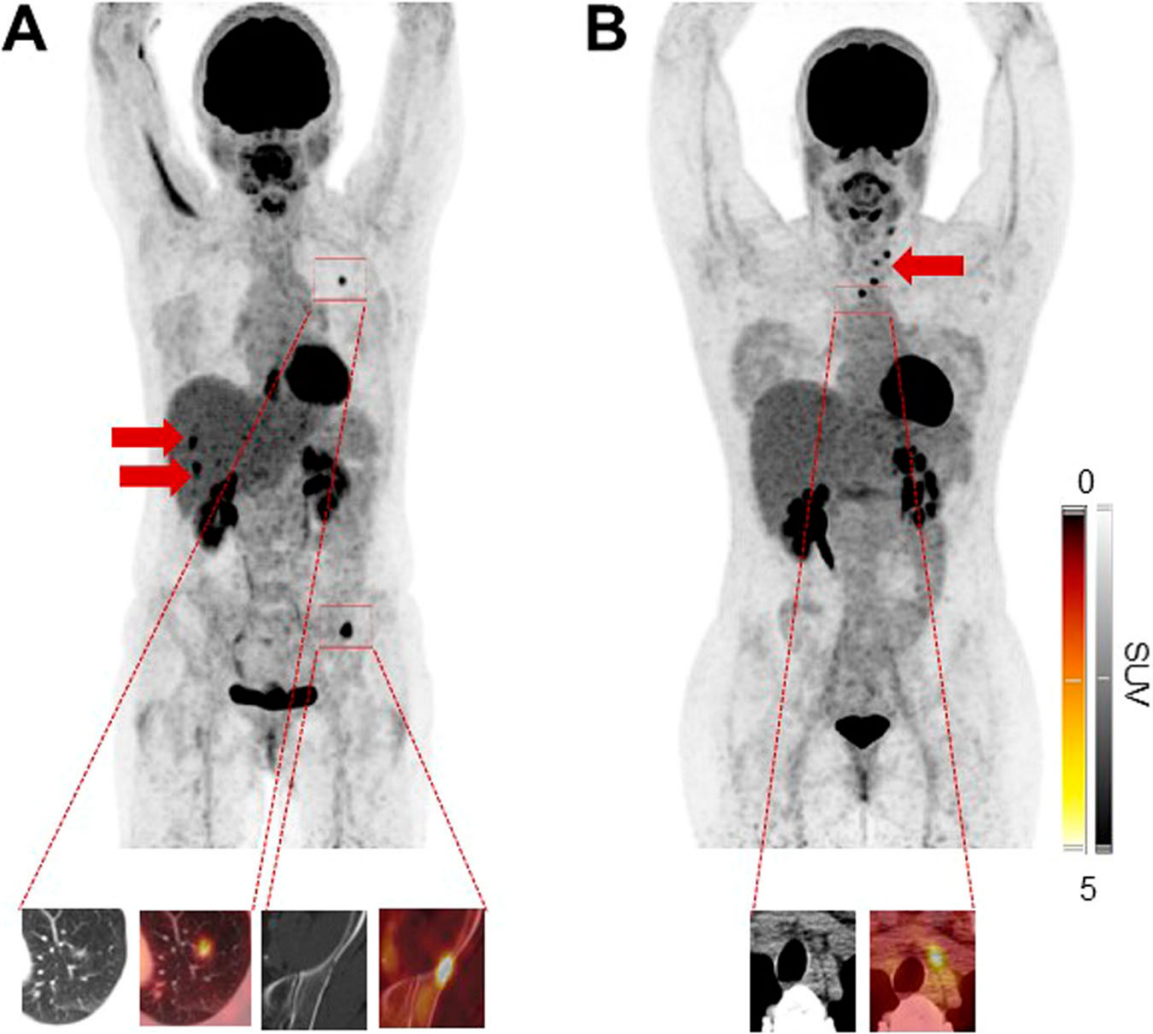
Patients with management changes after [^18^F]FDG PET/CT, with (A) systemic and (B) non-systemic treatment alterations. Maximum intensity projections and transaxial CT and PET/CT portions of target lesions are also displayed. Subject in A showed multiple organ involvement with PET-positive pulmonal and osseous findings (in the left pelvis) displayed on transaxial CT and PET/CT. Of note, lung lesions were only identifiable in the PET/CT images. Additional PET-positive hepatic lesions are not shown on transaxial slides, but seen on the MIP (arrows). Given widespread disease revealed by [^18^F]FDG, this patient was scheduled for tyrosine kinase inhibitor. Patient in B, however, only presented with cervical PET-positive lymph nodes (arrow, also seen on transaxial PET and PET/CT), triggering neck dissection of [^18^F]FDG-avid findings. Both patients then presented with controlled disease upon follow-up
